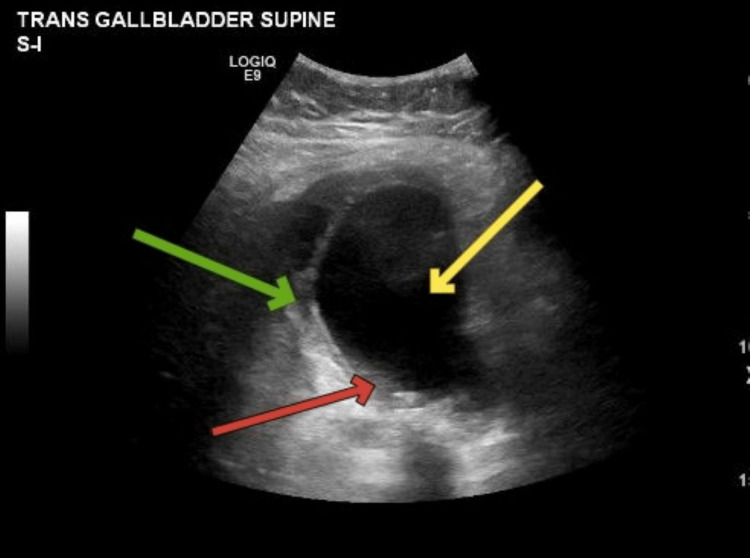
Ultrasound showing the gallbladder Ultrasound of the gallbladder showing a yellow arrow pointing at the distended gallbladder, a green arrow pointing at the gallbladder wall thickening with some pericholecystic fluid, and a red arrow pointing at the gallbladder stones.
